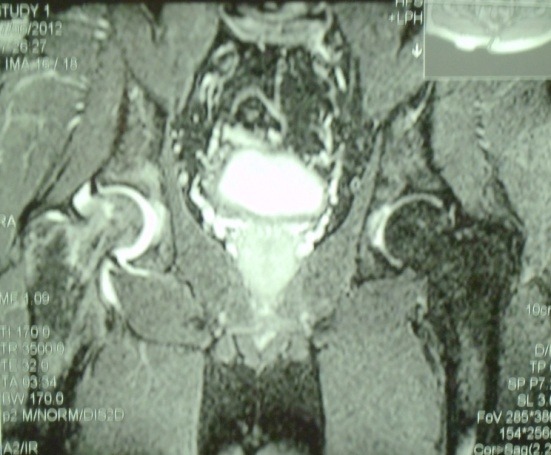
Avascular Necrosis in the right femoral head of a 23-year-old patient, MRI
